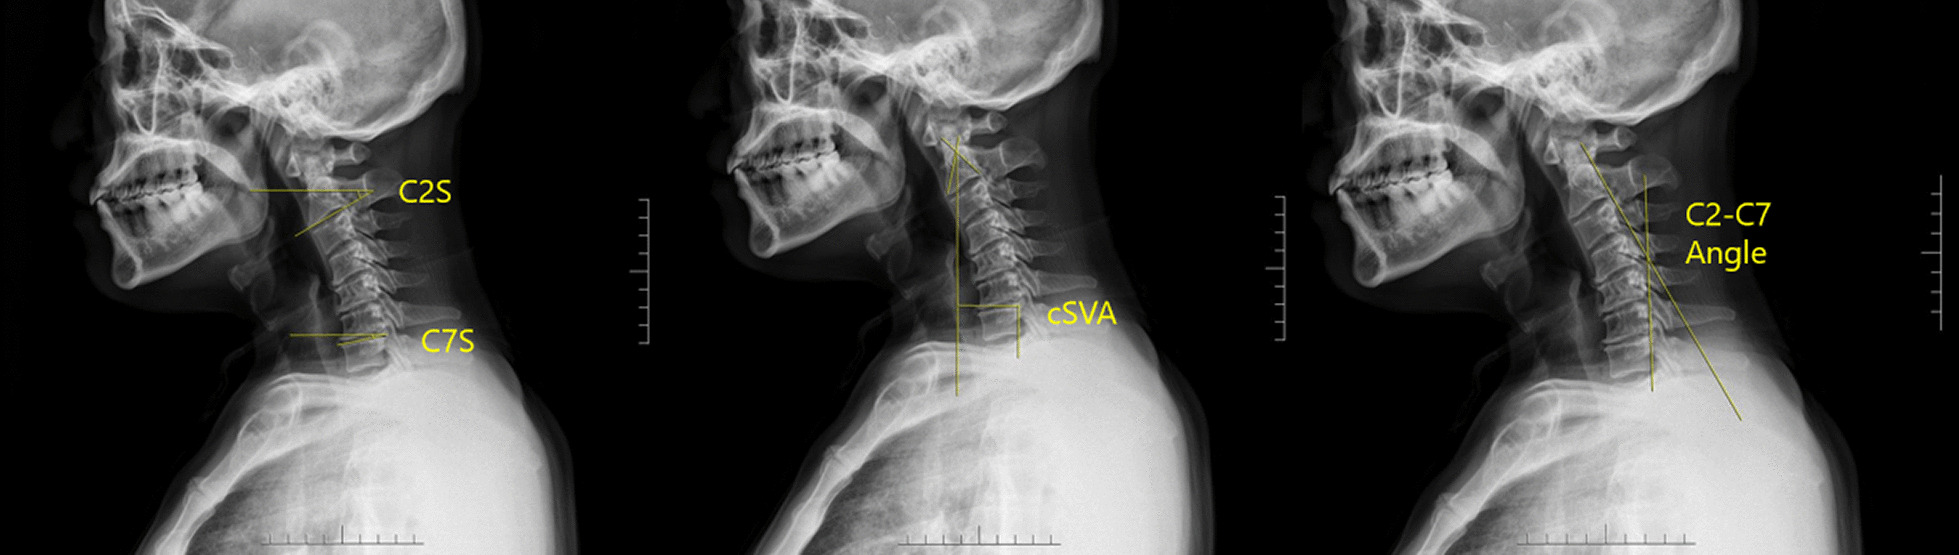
Various radiographic measurements used in this study: C2S, C7S, cSVA, and C2–C7 Angle
